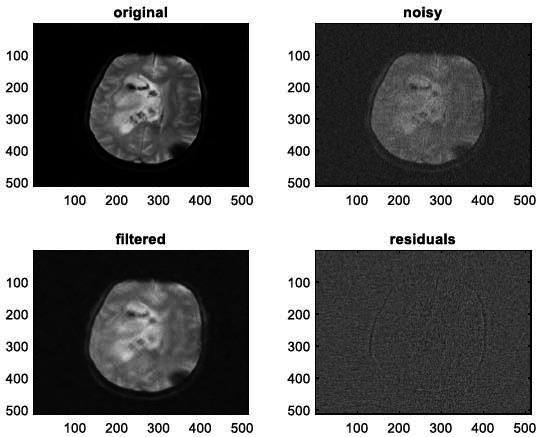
The fourth example of MRI denoising by applying the proposed image denoising technique.
